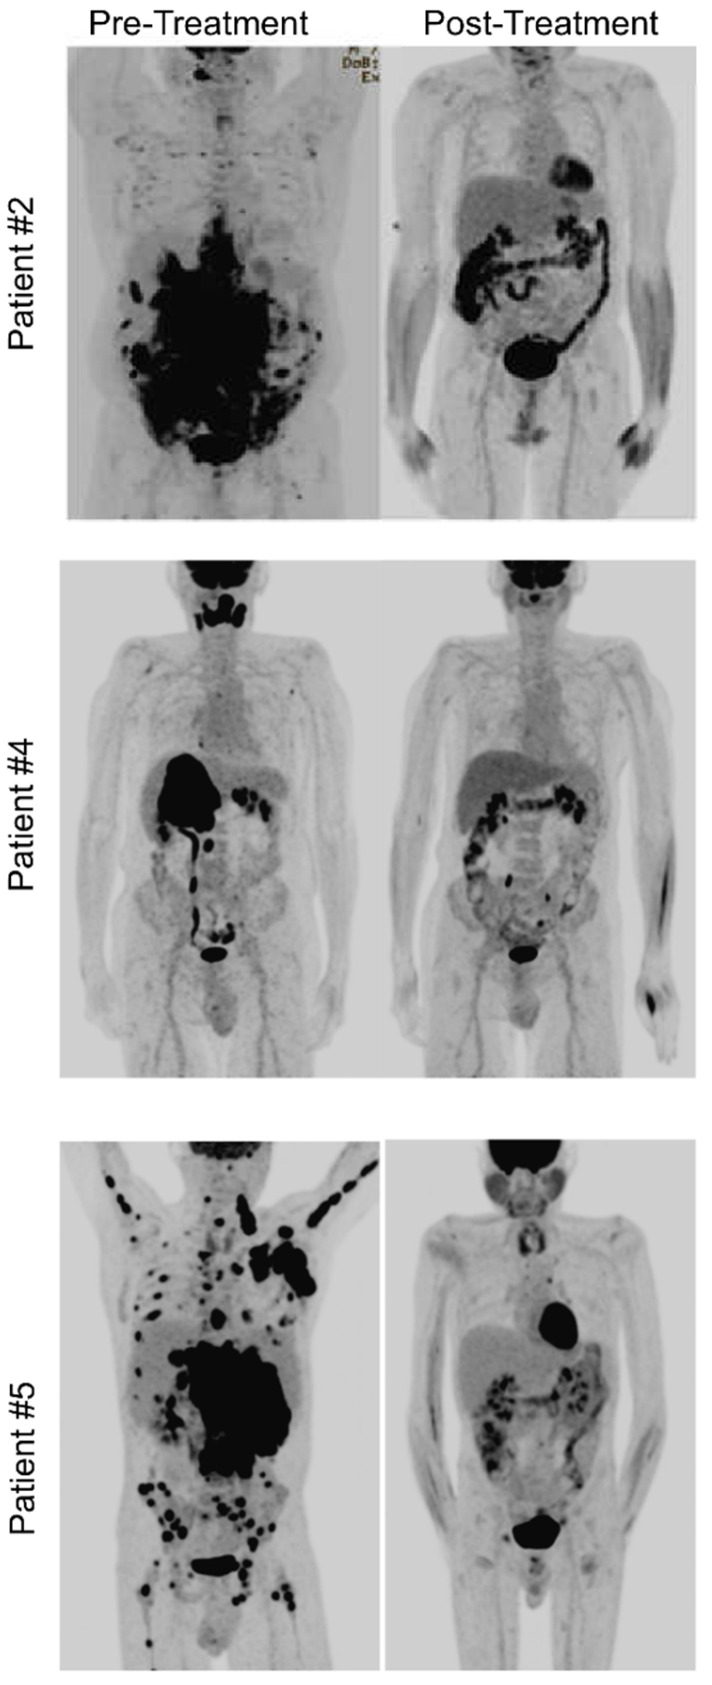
PET-CT of patients’ pre-treatment and post treatment. Patient 5 was thought to have PR at post-treatment, with residual R partially necrotic inguinal lymph node (2.3 × 1.9 cm, SUV max 4.5), but subsequent R inguinal lymph node biopsy demonstrated only a hernia sac and no evidence of lymphoma.
